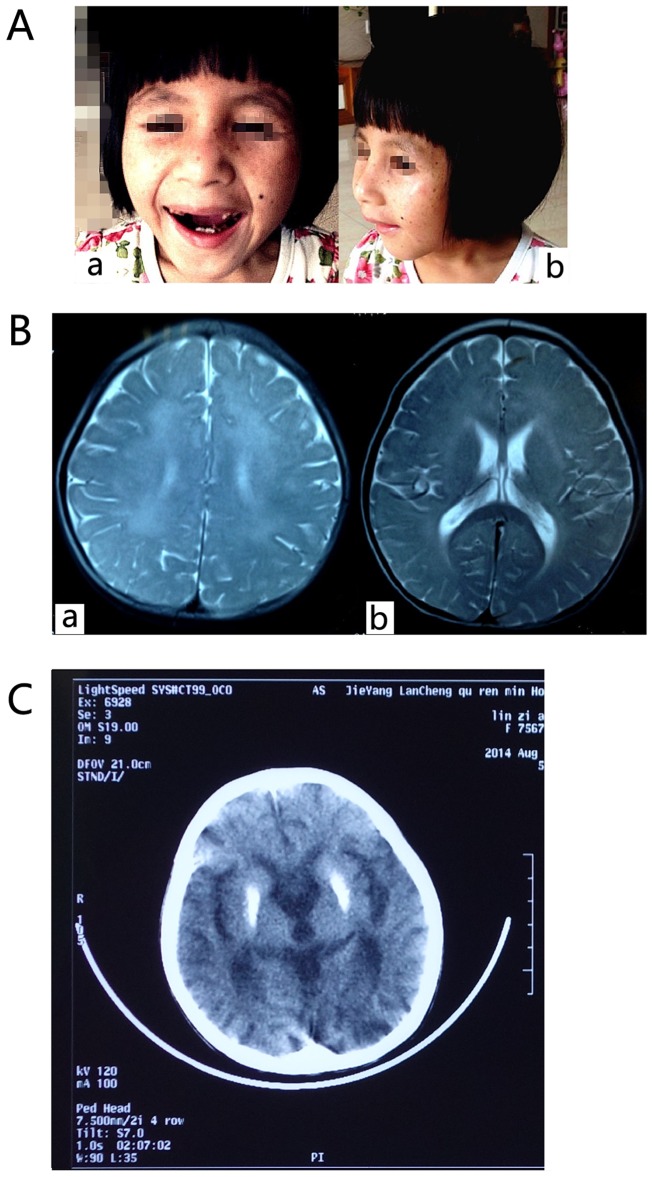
The face photo, skull MRI and CT scan of the proband. A) The face photo of the proband (a, the normal position; b, the lateral position). B) The skull MRI of the proband at 2 years old (a, the plain scanning; b, enhanced scanning). Hyperintensity was seen in centrum semiovale bilaterally, corona radiate, white matter of frontal and parietal lobe, anterior limb of internal capsule, external capsule in T2 weighted images. T2 weighted images show less low signal in the posterior limb of internal capsule. C) The brain CT scan shown that calcification on bilateral globus pallidus, cerebral atrophy (widened sulcus and cleft, enlarged superatentorial ventricle, narrowed gyrus).
